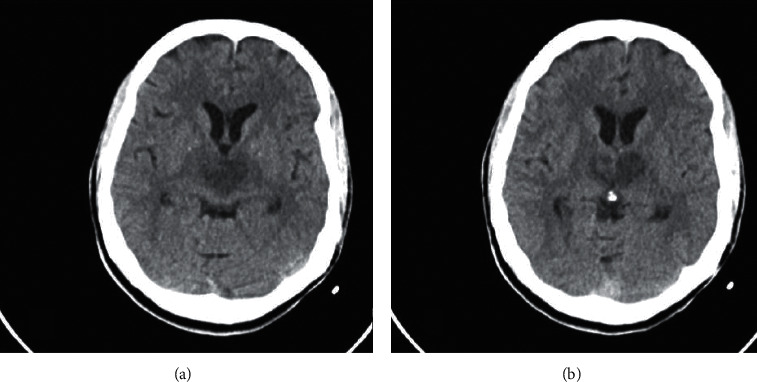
Noncontrast CT brain demonstrating bilateral hypodensities in the medial thalami obtained at the time of admission.
